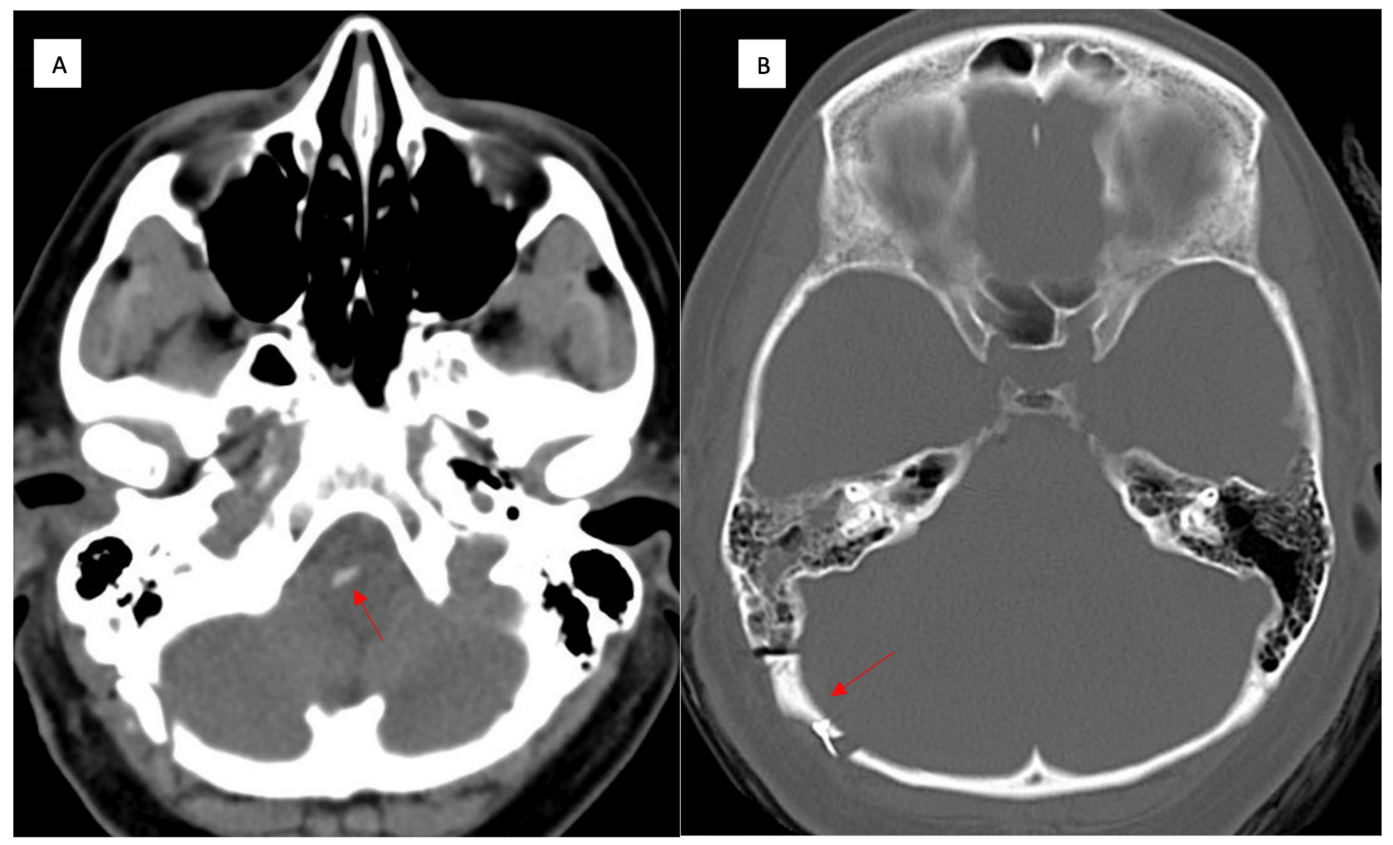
Postoperative computed tomography (CT) scan performed one day after surgery Image A axial view shows the position of the pledget next to the medulla on the right (arrow). Image B is the same CT scan with bone windowing at a more cephalad level and shows the position of the craniotomy (arrow) and opening into the mastoid air cells.
